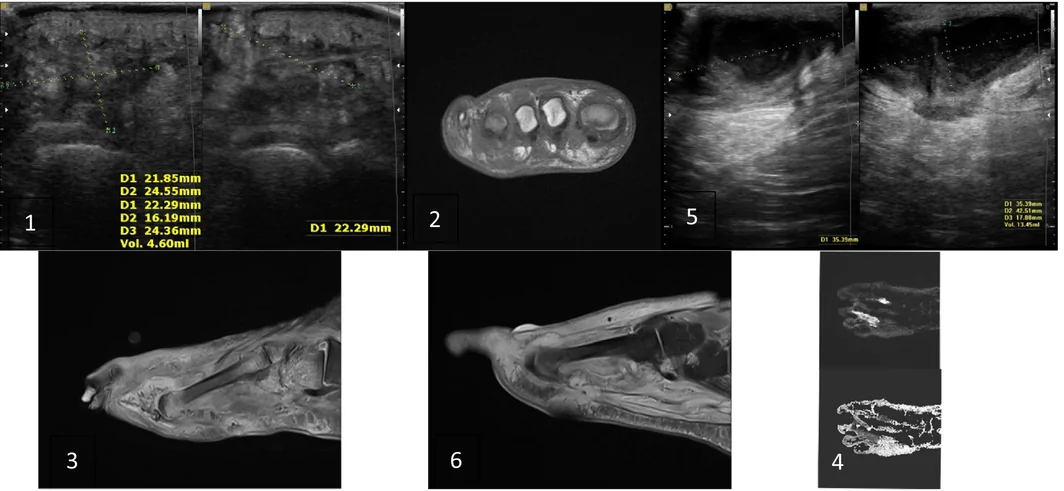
Multimodal imaging of DF infection in the same patients. (1) Ultrasound demonstrates a hypoechoic soft tissue collection consistent with an abscess. (2) Axial T1‐weighted MRI reveals OM involving the fourth metatarsal head. (3) Sagittal proton density (PD) sequence shows a plantar skin defect at the metatarsophalangeal (MTP) joint with an adjacent soft tissue collection. (4) Axial diffusion‐weighted imaging (DWI, *b* = 800) and corresponding apparent diffusion coefficient (ADC) map highlight a diffusion‐restricted collection in the forefoot, suggestive of an abscess. (5) There is increased echogenicity of subcutaneous fat and fluid separating fat lobules, suggestive of cellulitis on US. Also, a fluid collection (abscess) is seen. (6) Sagittal PD fat‐sup MRI of the foot revealed OM in the head of the 3rd metatarsal bone, surrounding an air‐containing collection.
